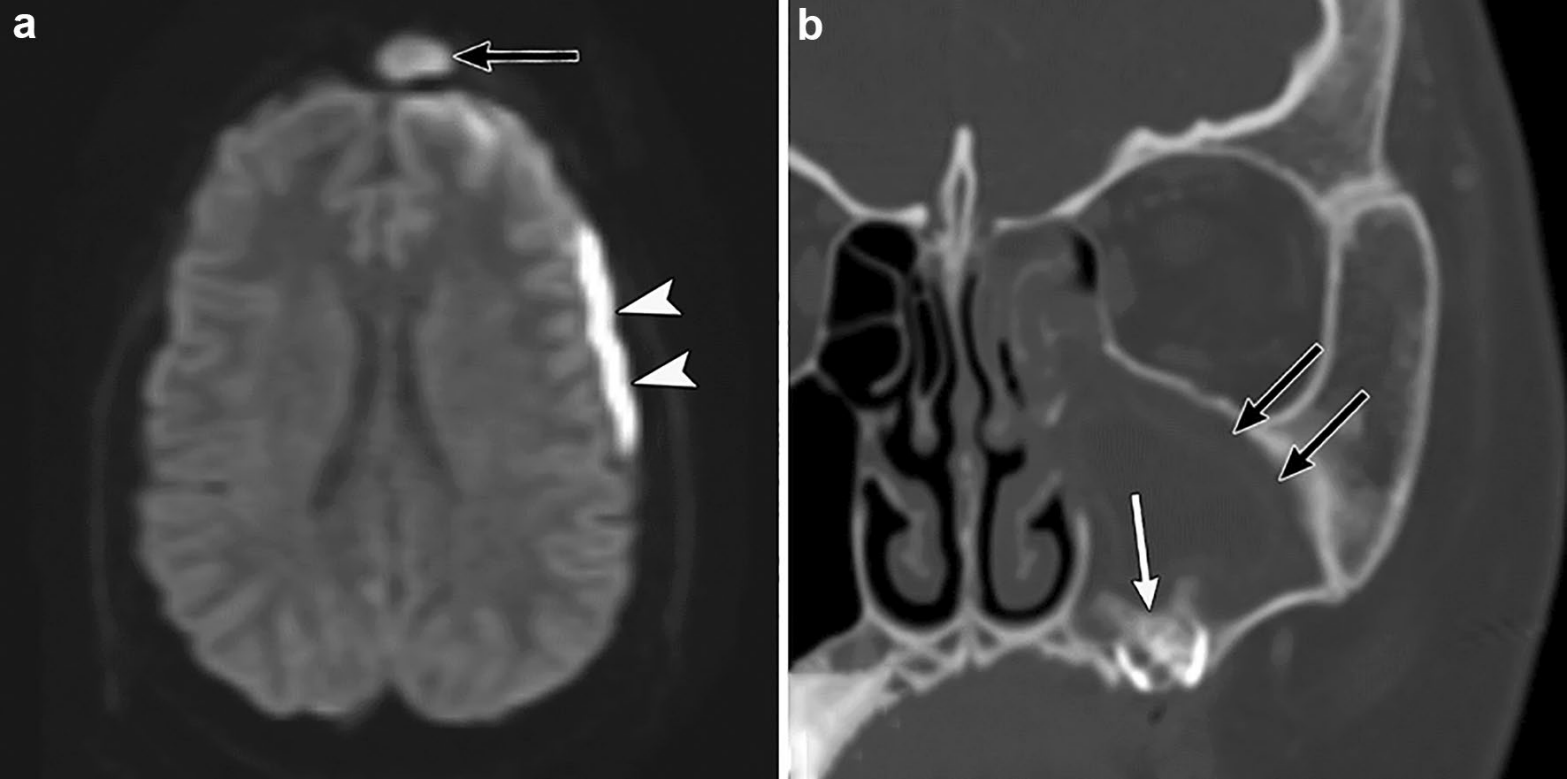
Odontogenic sinusitis in a 12-year-old girl with bacterial meningitis. a Diffusion-weighted image shows subdural fluid accumulation with diffusion restriction, suggestive of subdural empyema (arrowheads). Restricted diffusion is also observed in the frontal sinus (black arrow). b Coronal computed tomography (bone window) shows the left maxillary sinus with massive fluid accumulation and mucosal thickening (black arrows). Impacted decaying teeth are observed in the left maxillary sinus (white arrow), confirming the diagnosis of odontogenic sinusitis. Surgical drainage for subdural empyema and endoscopic sinus surgery were performed. The impacted teeth were extracted
